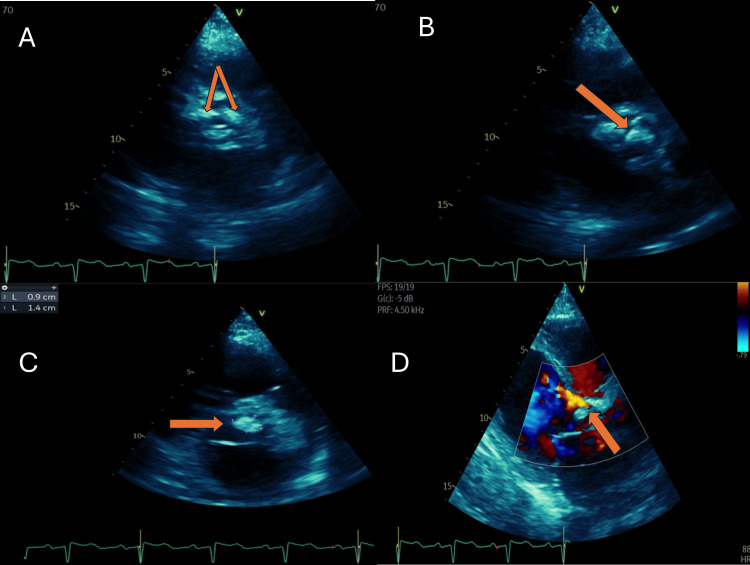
Transthoracic echocardiogram (A) Parasternal short-axis view at the level of the great vessels, demonstrating the aortic valve at the center of the image. A bicuspid aortic valve is identified, with fusion of the right and left commissures, thickened leaflets, and significant calcification. Orange arrows indicate the thickened and calcified valve leaflets. (B) Parasternal long-axis view, demonstrating the aortic valve with a hyperechoic mobile structure suggestive of vegetation. The orange arrow indicates the echogenic mass attached to the aortic valve leaflet, consistent with infectious vegetation. (C) Parasternal long-axis view with measurement of the vegetation, demonstrating dimensions of 0.9 × 1.4 cm. The orange arrow indicates the vegetation on the aortic valve. (D) Color Doppler demonstrating an eccentric aortic regurgitation jet originating at the level of the aortic valve. The turbulent, mosaic color pattern is consistent with severe aortic regurgitation secondary to valvular destruction. The orange arrow indicates the eccentric regurgitant jet extending into the left ventricular outflow tract.
